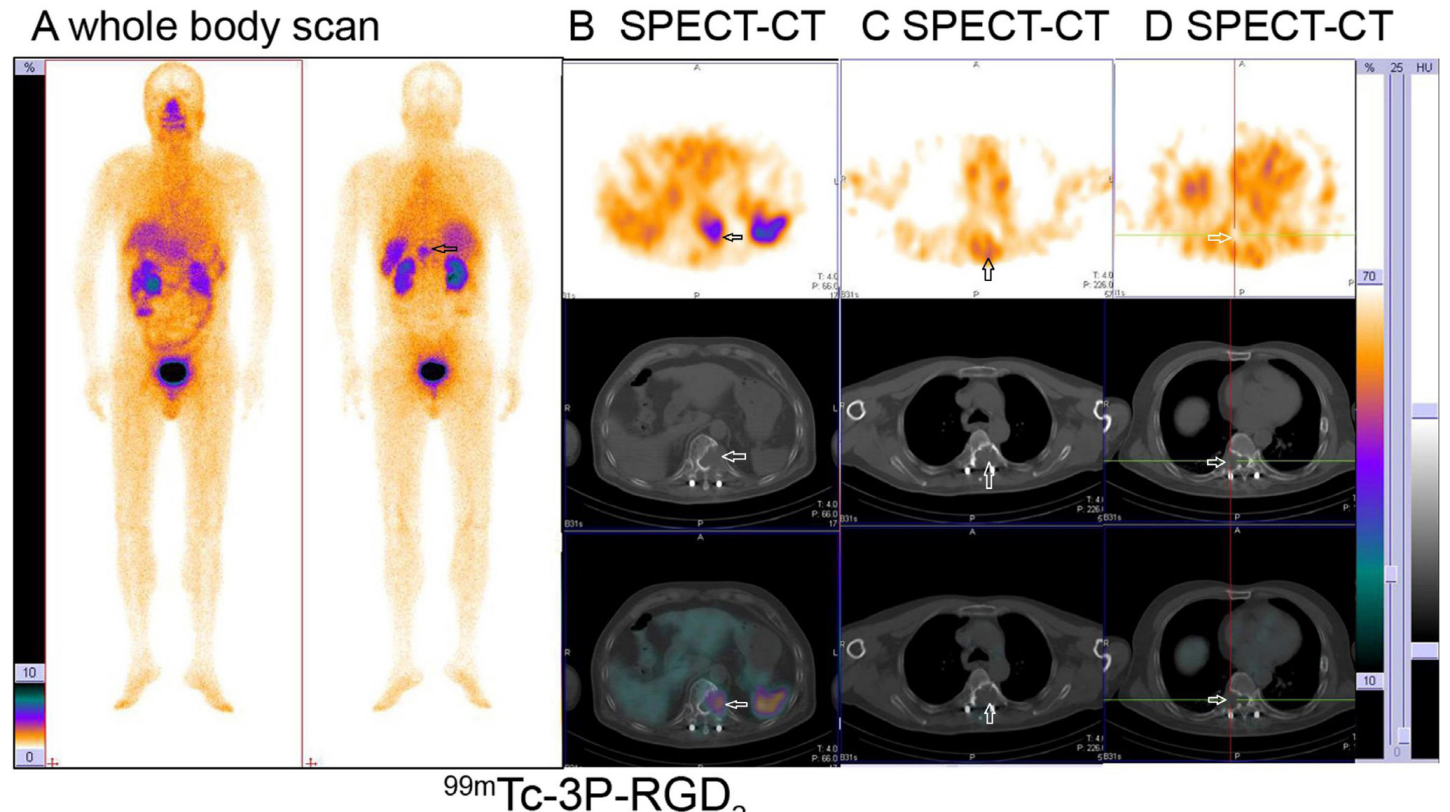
Osteolytic bone metastases from lung cancer displayed as significant accumulation (hot area) (A, B), slight higher uptake (C) or absence (cold area) (D) of ^99m^Tc-3P-RGD_2_ on whole body planar scan (A) and SPECT-CT (B, C, D) with bone destruction on CT (see arrows)
